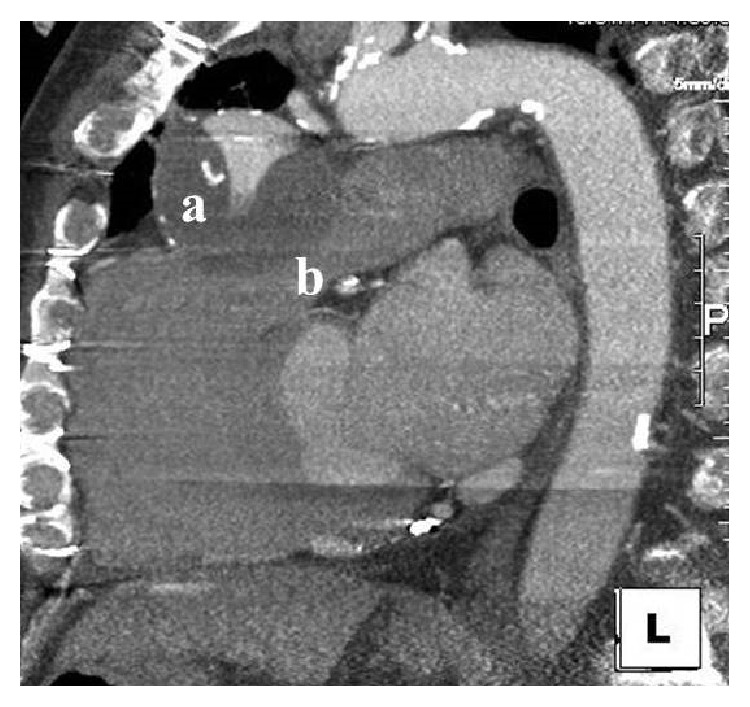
Coronary CT angiogram showing giant saphenous venous graft aneurysm (a) compressing the pulmonary artery (b).
